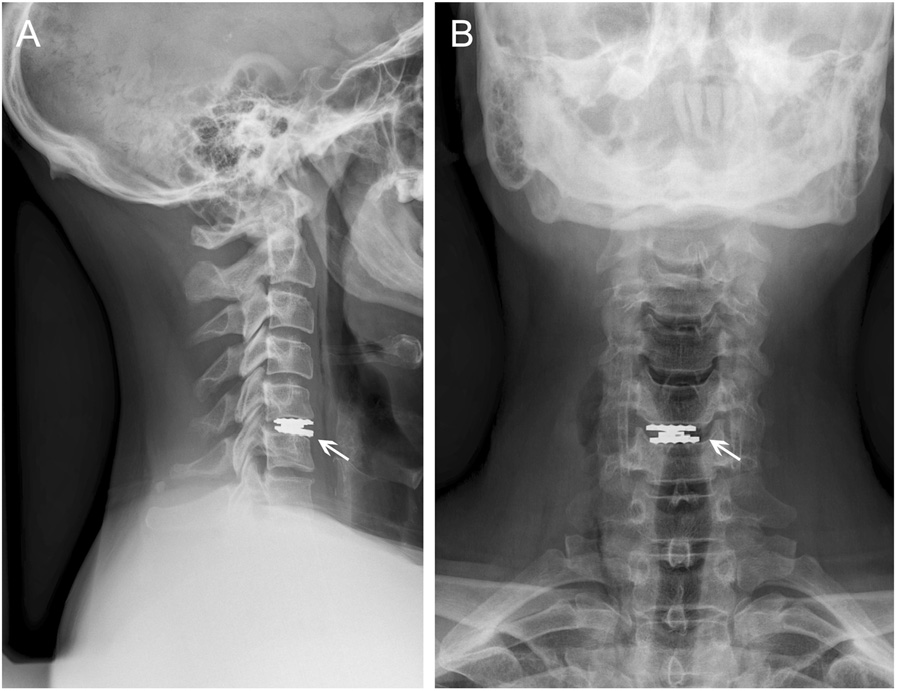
X-ray in neutral position (a: lateral view; b: anterior view) showing an undersized and too ventrally positioned ROTAIO cervical disc prosthesis at the level C5-C6 (white arrows) in a 38 years old male patient, 3 month after implantation. Within the first 12 month of follow up the patient suffered from progressive neck pain
